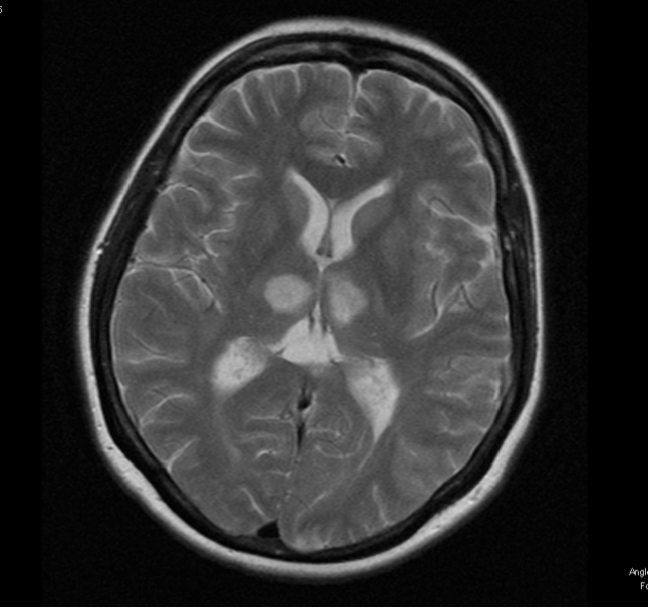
MRI Head showing bilateral symmetrical lesions in both thalami giving high signal changes on T2 weighted images.
